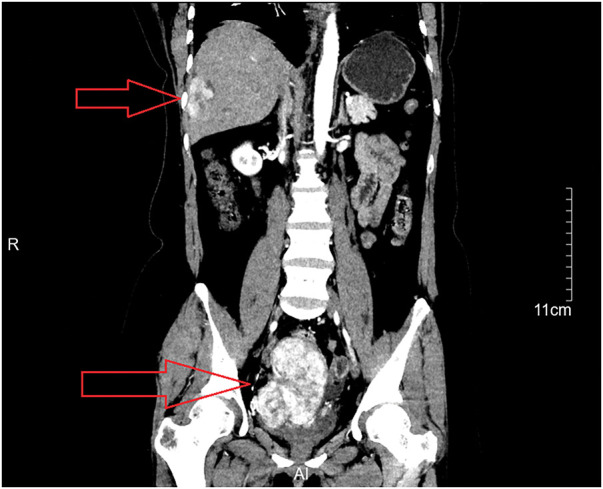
CT coronal view shows a pelvic tumor and subphrenic metastases.
